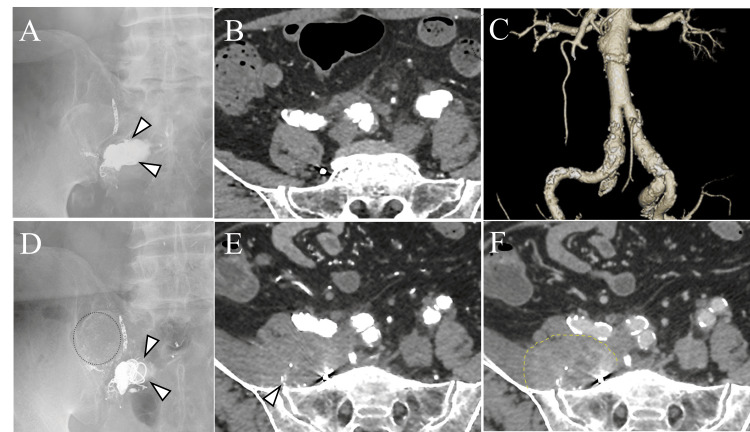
Postoperative images (A-C) An abdominal radiograph and contrast-enhanced CT obtained shortly after the embolization of the right internal iliac artery aneurysm and open AAA repair showed no migration of NBCA mixed with lipiodol (arrowheads). The shape of the coils and blood vessels is unchanged, and the AAA is repaired. (B) Arterial phase. (C) Volume–rendering image. (D) An abdominal radiograph obtained five months postoperatively shows the migration of the NBCA-lipiodol mixture cast around the coils (dotted line, arrowheads). (E, F) Abdominal contrast-enhanced CT obtained at 12 months postoperatively shows the appearance of a 70-mm irregular mass in the right retroperitoneum surrounding the iliopsoas muscle (dotted line). The lesion contains multiple, small, hyperdense areas suggested to be extravascular migration of NBCA-lipiodol mixture casts (arrowhead). The lesion had mild enhancement in the venous phase. (E) Arterial phase. (F) Venous phase.
